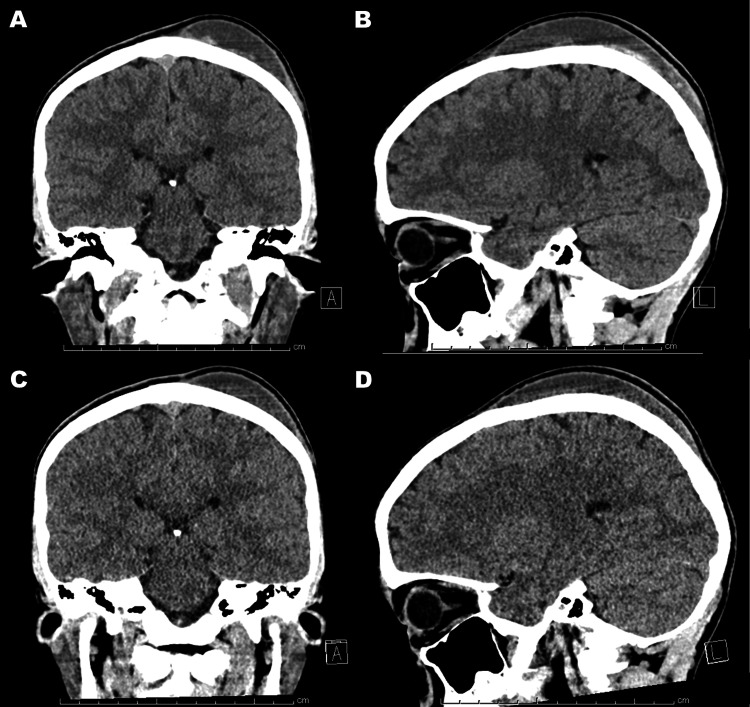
CT scan images before a simple needle aspiration and after five days. A simple needle aspiration of the C-SPOH (A, B) fails to cure, and accumulation is allowed again (C, D). C-SPOH, calvarium subperiosteal hematoma
